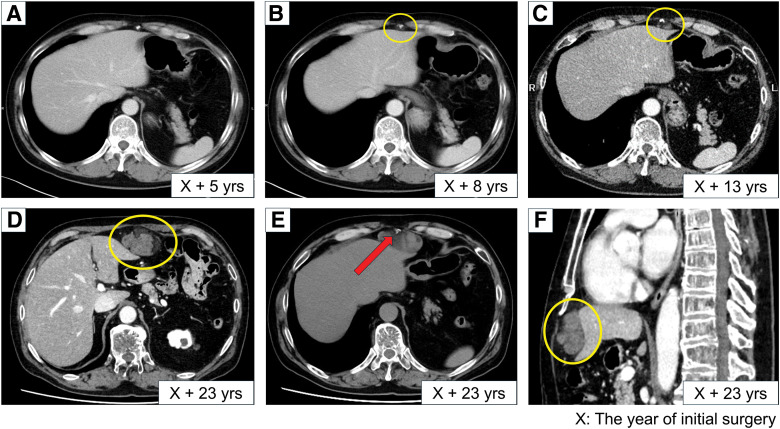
CT findings. (A) Five years after the initial surgery, no lesion was identified in the subxiphoid region. (B) Eight years after the initial surgery, a retrospective review revealed a very small area of increased fat attenuation in the subxiphoid region (yellow circle). (C) Thirteen years after the initial surgery, a retrospective review demonstrated a slight increase in the size of the subxiphoid lesion (yellow circle). (D) Twenty-three years after the initial surgery, the lesion had clearly enlarged (yellow circle). (E) Calcified components were observed within the lesion (red arrow). (F) A sagittal CT image obtained 23 years after the initial surgery, demonstrating the lesion in the subxiphoid region (yellow circle).
